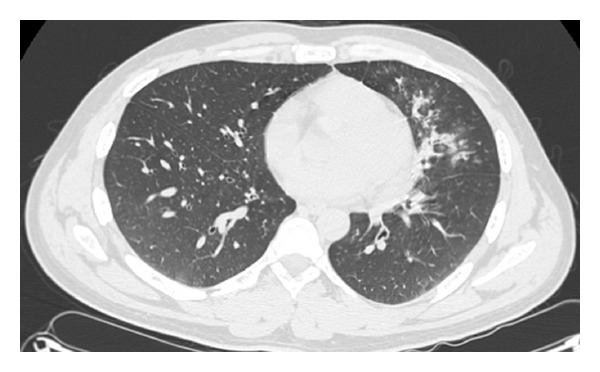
CT Chest of the first patient showing diffuse interstitial thickening, patchy infiltrates and areas of consolidation of the left lower lobe.
